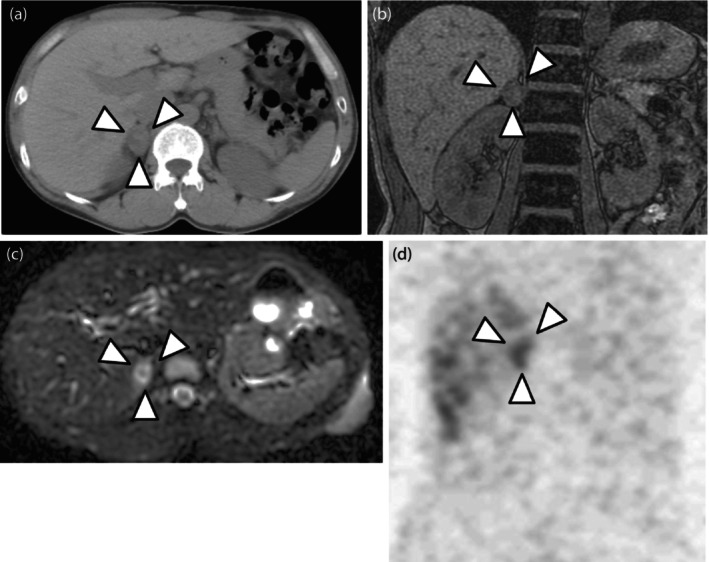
(a) CT shows a right adrenal tumor that is 2.8 cm in diameter. (b) MRI shows a hypointense mass on T1 weighted images in the right adrenal gland. (c) Diffusion‐weighted images show a heterogeneous tumor in the same region. (d) ^123^I‐MIBG scintigraphy confirming a localized increased uptake in the same region.
